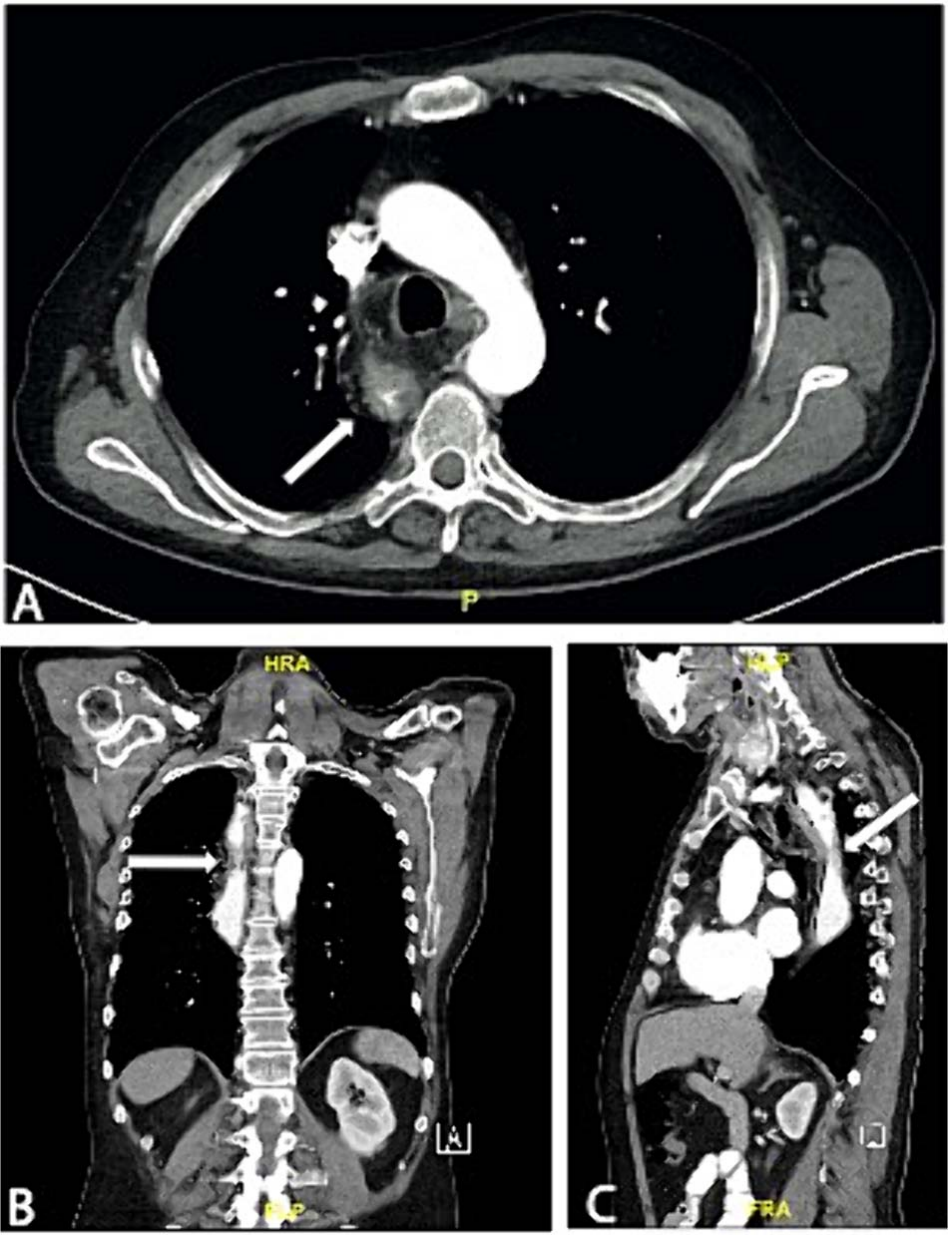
Axial (A), coronal (B), and sagittal slice (C) of a preoperative CT scan showing wall thickening of the neoesophagus proximal to the tracheal bifurcation.
